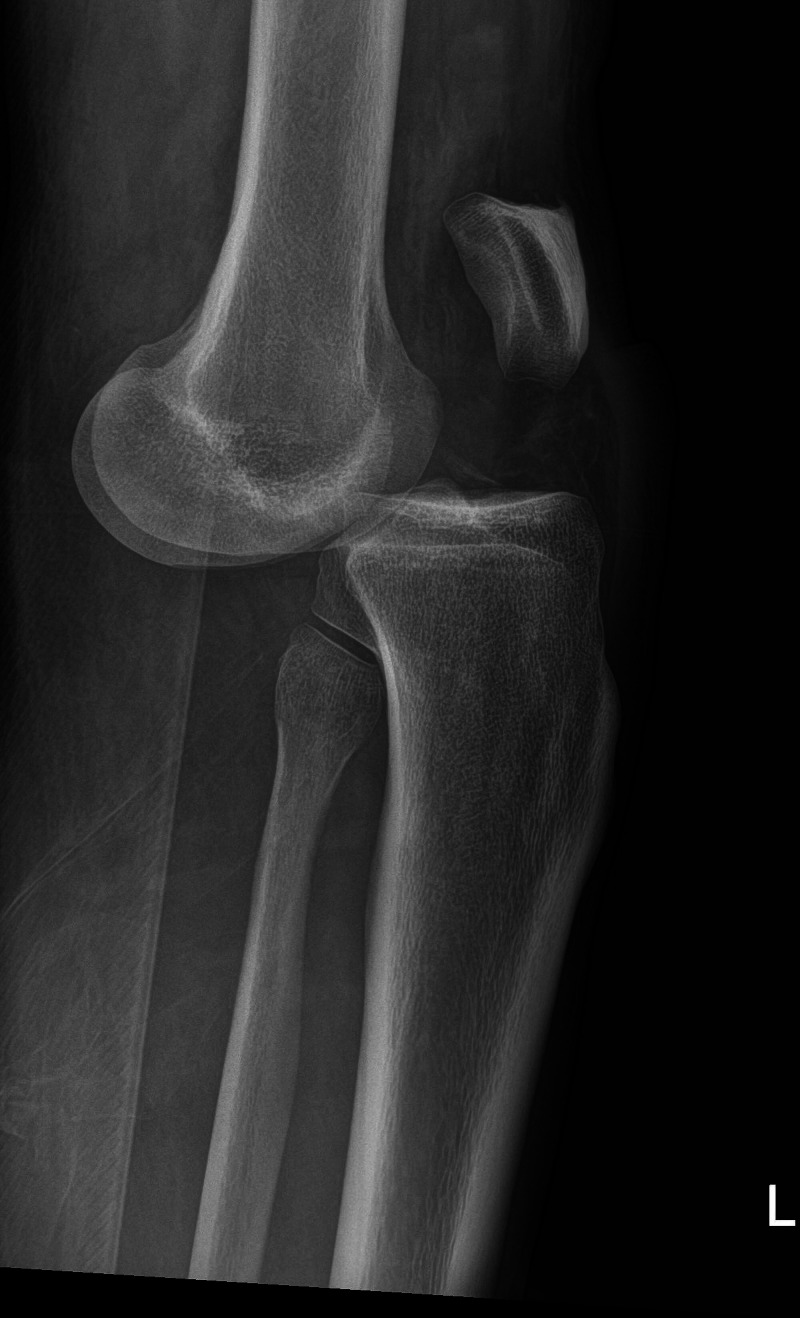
Lateral radiograph of the left knee in patient 2 Lateral radiograph of the left knee demonstrating acute anterior dislocation.
